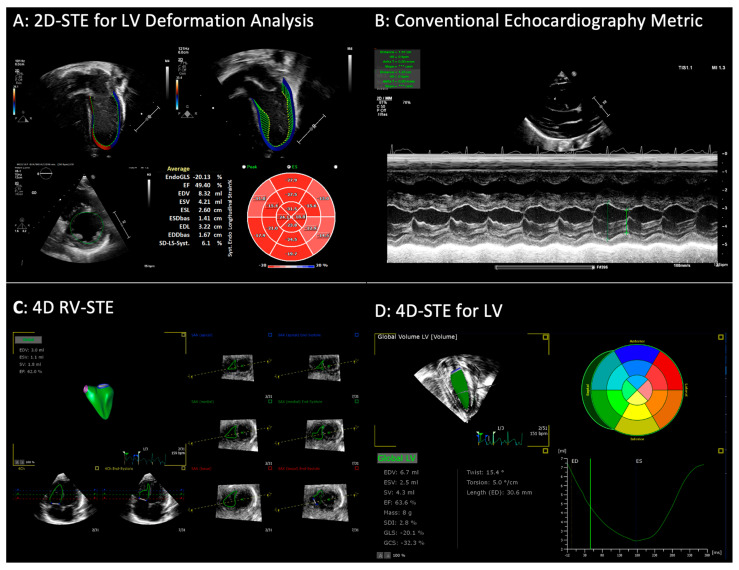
Sample echocardiographic images with 2D and 4D speckle tracking of LV and RV. Abbreviations: 2D (bi-dimensional); 4D (four-dimensional); LV (left ventricle); RV (right ventricle); MMode (motion-mode); STE (speckle tracking echocardiography).
